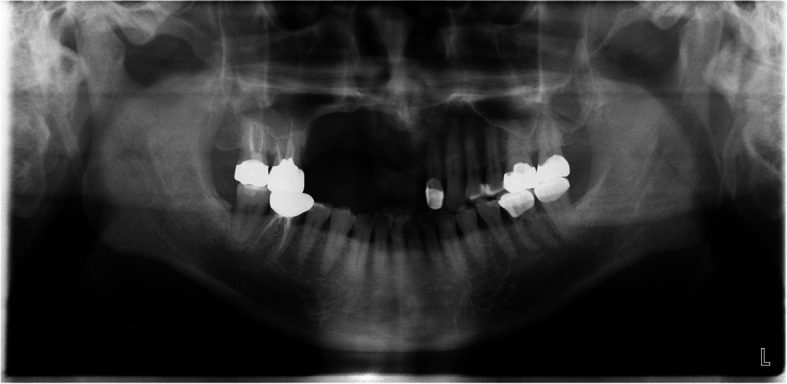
Panoramic radiograph at 2 years and 7 months postoperatively shows no evidence of recurrence
